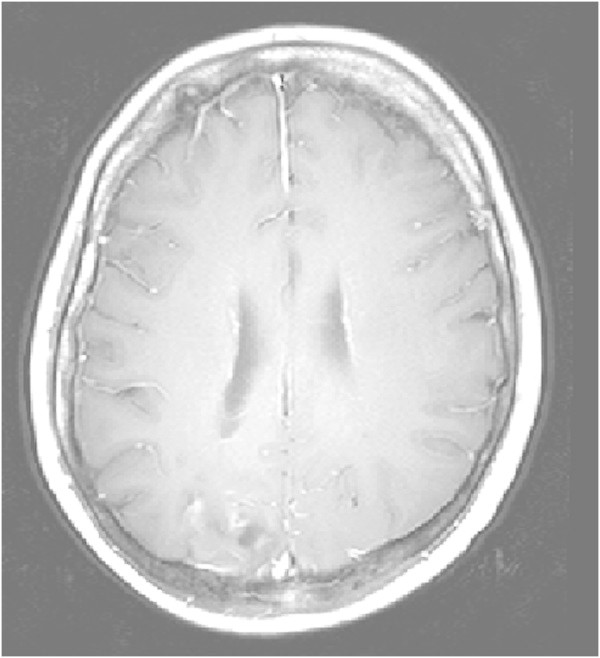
MRI after 5 months, transverse T1-weighted image after contrast injection.
